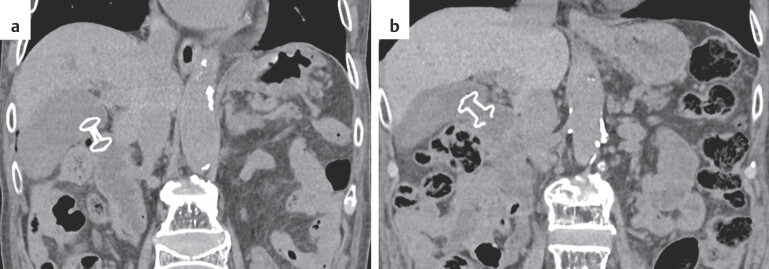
Computed tomography after endoscopic ultrasound-guided
